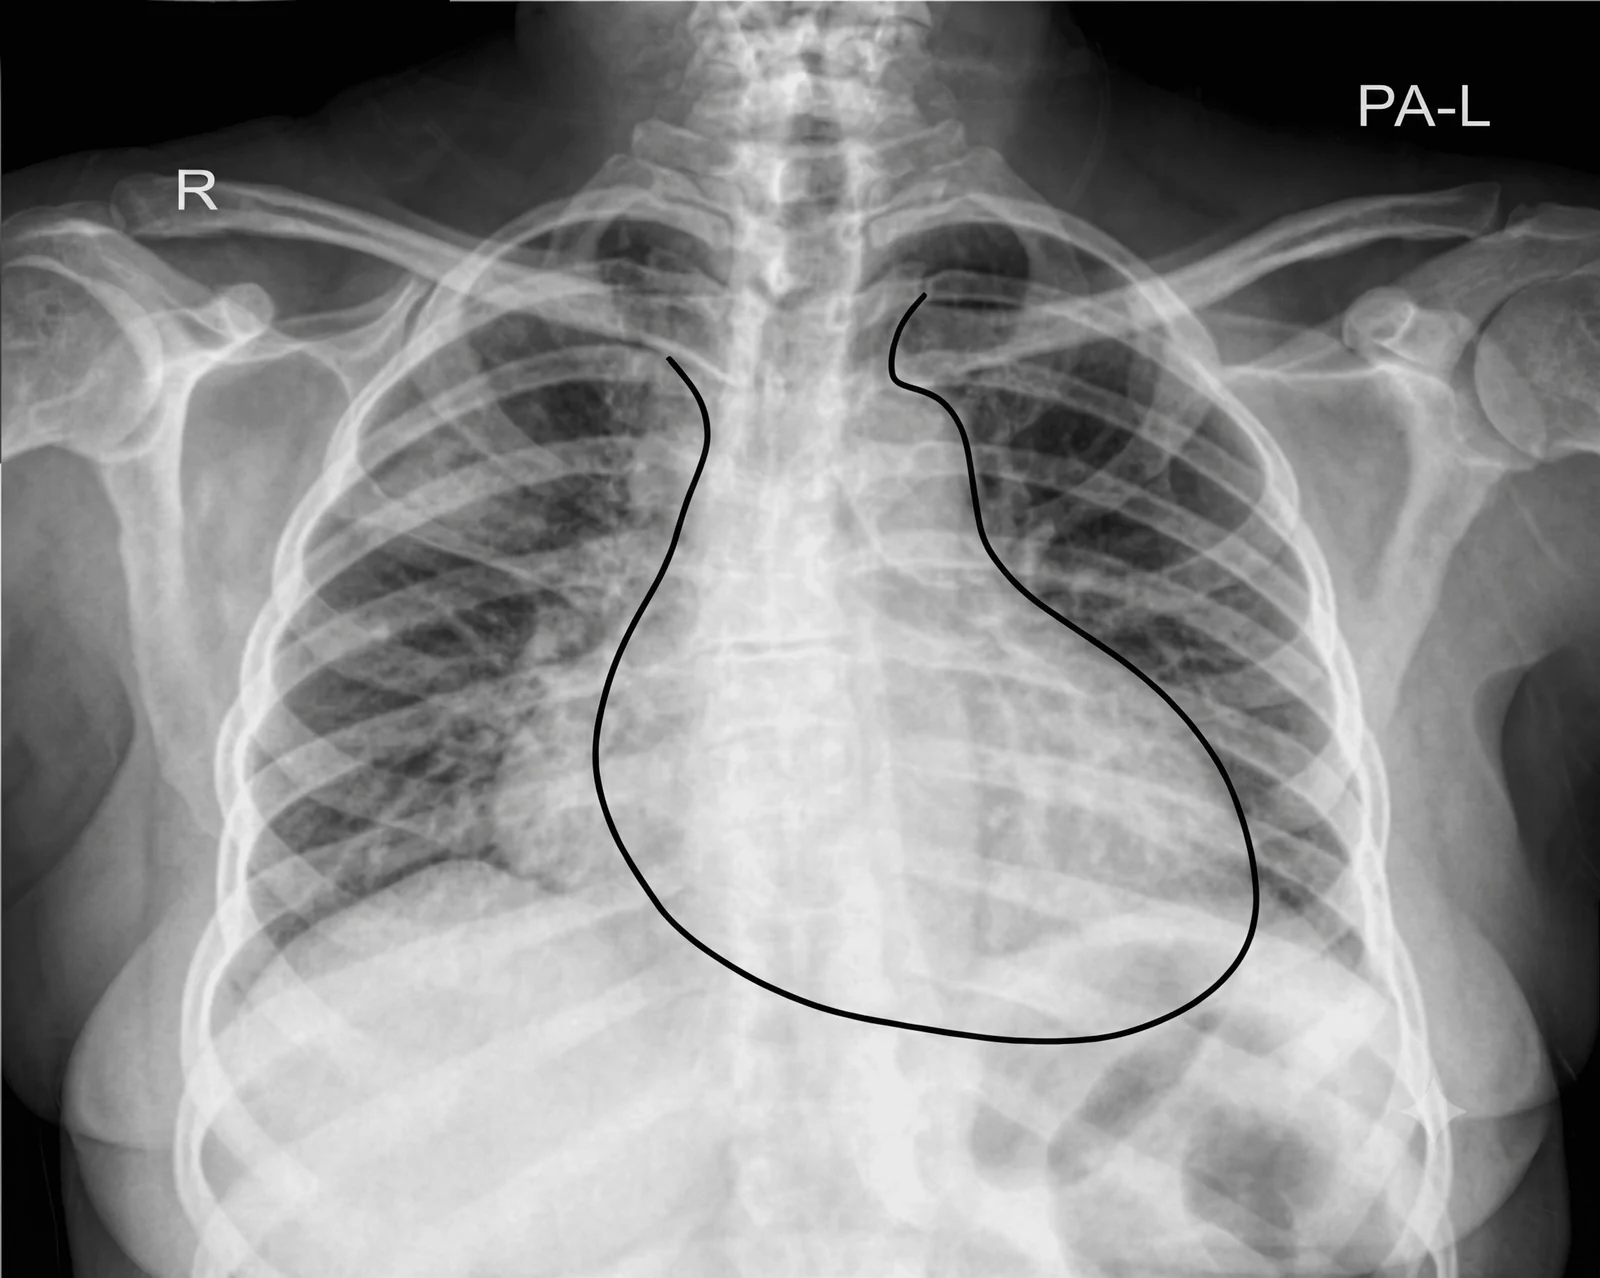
Chest radiograph. Cardiomegaly (enlarged heart outlined in black). No nodular opacities or pulmonary consolidations.
